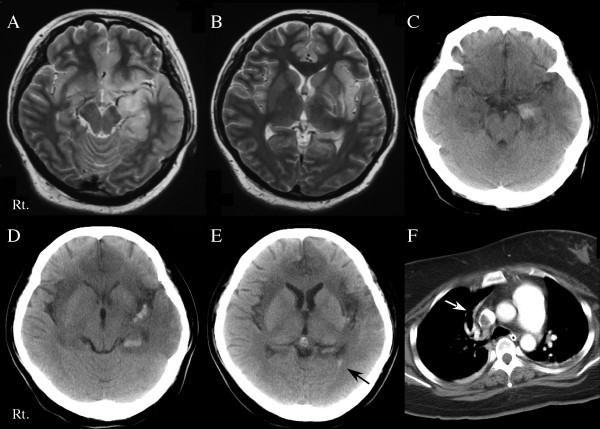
Cranial T2-weighted magnetic resonance imaging (panel A and B) showed left-predominant bilateral regions of increased signal intensity in the hippocampus and amygdaloid body, the insular, medial temporal and medial frontal lobes. Cranial computed tomography (CT; panel C) demonstrated high intensity lesions in the left amygdaloid body. Subarachnoid and intraventricular bleeding, in addition to low intensity lesions in the bilateral frontal and temporal lobes, was evident (panels D and E). A chest-enhanced CT demonstrated massive thrombosis of the right pulmonary artery trunk (panel F).
